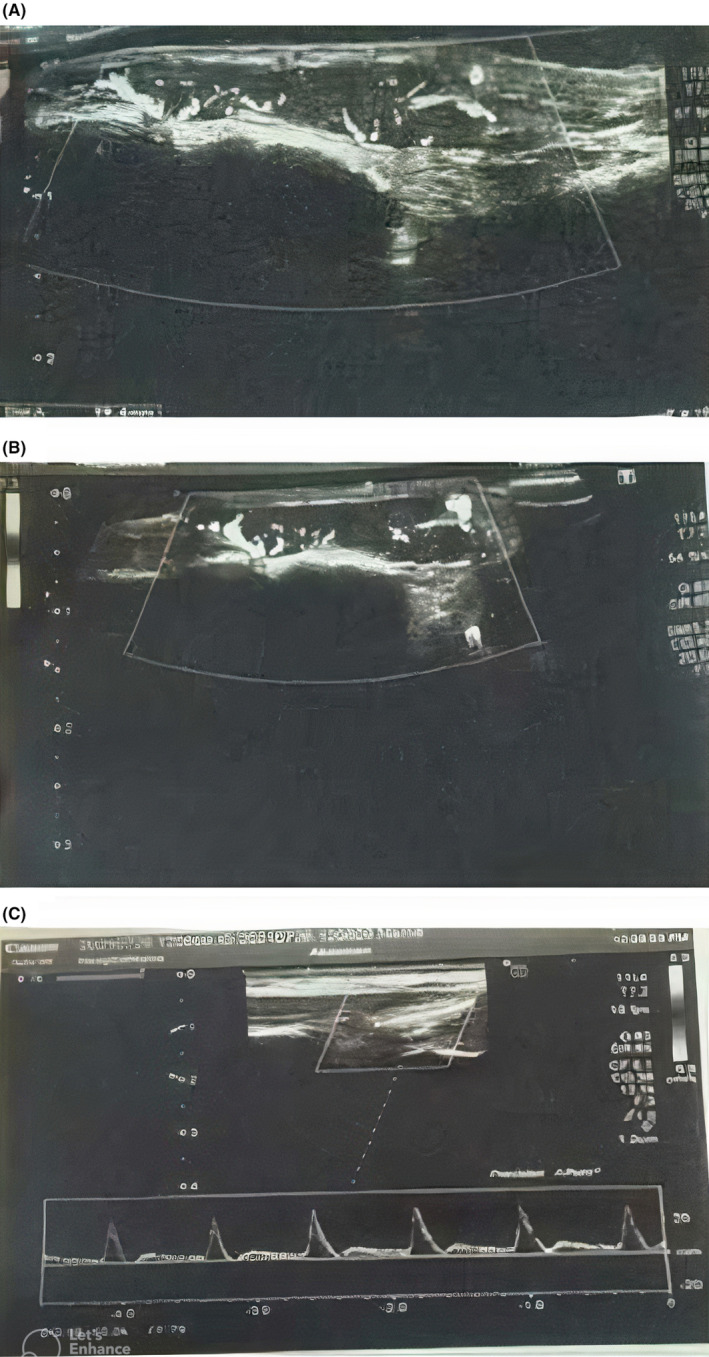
Lesion ultrasound findings showcase a 23 × 5 mm subdermal hypoechoic area over the fourth tarsometatarsal joint, featuring vascularity within and around the lesion, and involvement of the adjacent tendon, with no signs of liquefaction or calcification.
